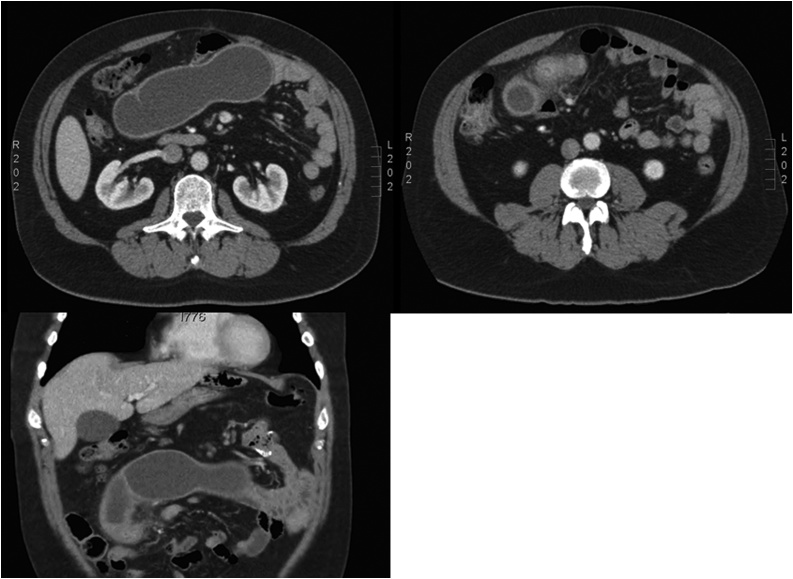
Contrast-enhanced CT-scan shows a 17 cm-long blind tubular lesion, filled with liquid contents and inflammatory changes around the base, located at proximal ileum.
